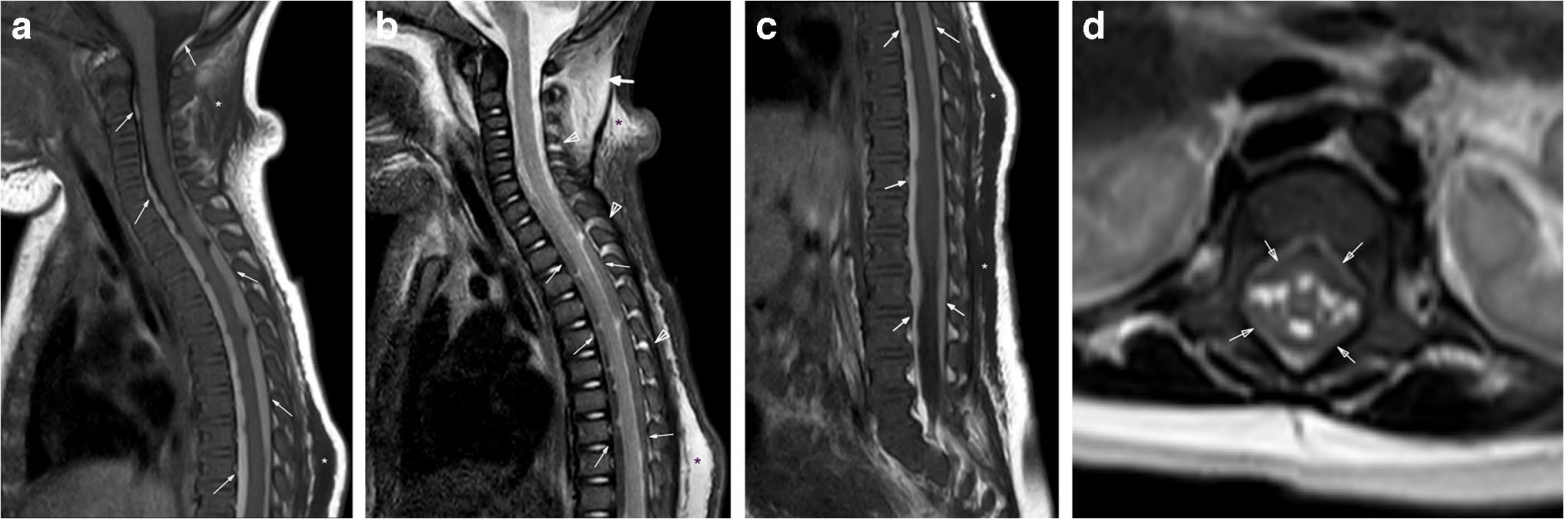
Four-month-old girl presenting with vomiting and seizure. Sagittal T1-weighted MR image (a) of the upper spine shows the hyperintense subdural hemorrhage (*arrows*) extending below along the spinal canal and nuchal ligament edema (*asterisk*). Sagittal STIR MR image (b) of the upper spine better demonstrates the dorsal soft tissue (*asterisk*) and ligamentous edema in the nuchal ligament (*thick arrow*), as well as interspinous ligamentous edema/injury (*arrowheads*). Note the STIR hypointense spinal SDH extending inferiorly from the level of CCJ with multiple loculations (*thin arrows*). Sagittal T1-weighted MR image (c) of the lower spine shows hyperintense subdural hemorrhage along the spinal canal (*arrows*). Note posterior subcutaneous soft tissue edema (*asterisk*). Axial T2-weighted MR image (d) from L1 level reveals the T2-hypointense subdural hemorrhage located at the anterior and posterior aspect of the spinal canal (*white arrows*)
